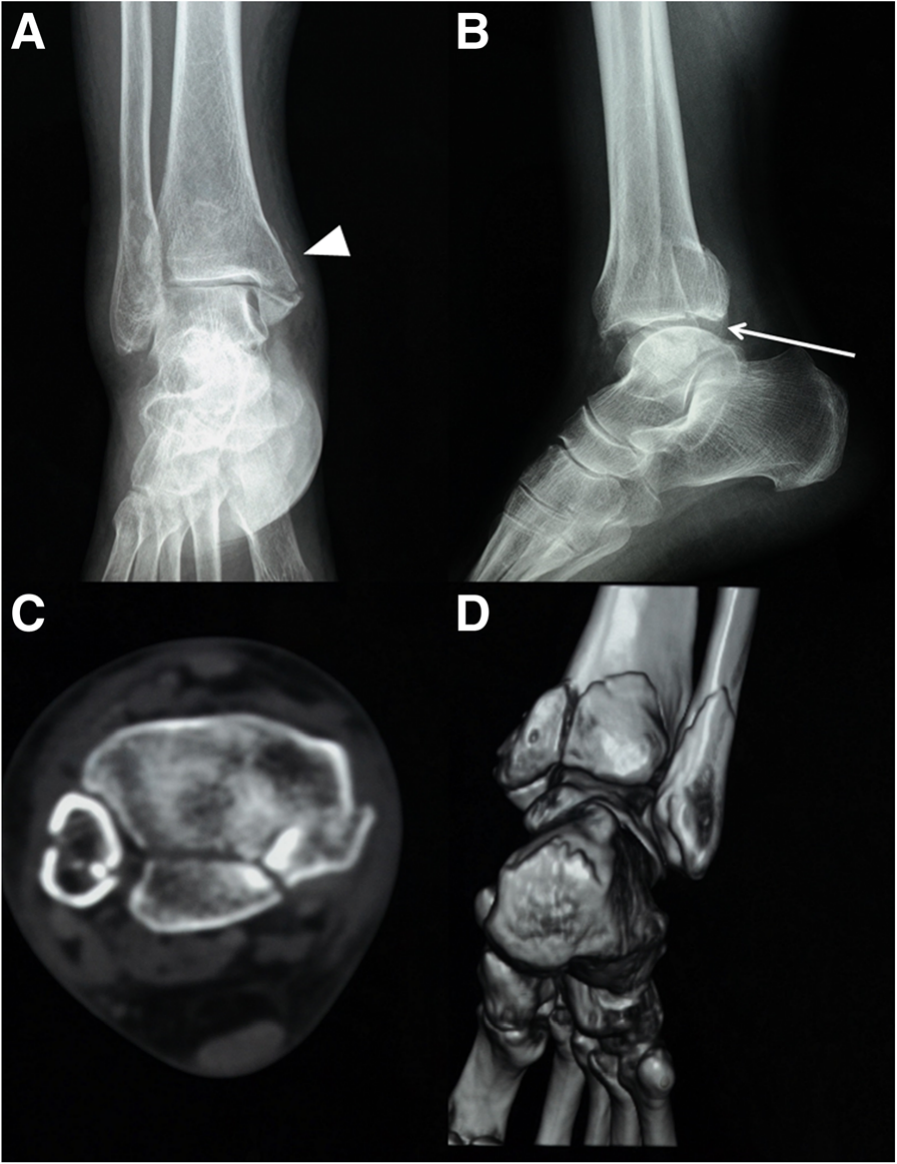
Preoperative radiographs of case 3. a-b The *Arrow Head* showing the “double contour” sign on AP view indicates the existence of PM fragment. The *Arrow* showing the “double joint line” sign on lateral view indicates the proximally displaced posterior tibial plafond. In together with the two signs, posterior pilon fracture is in highly suspicion. c-d CT scan and reconstruction of the same patient provides a better view of the location and size of the PM, PL fragment
